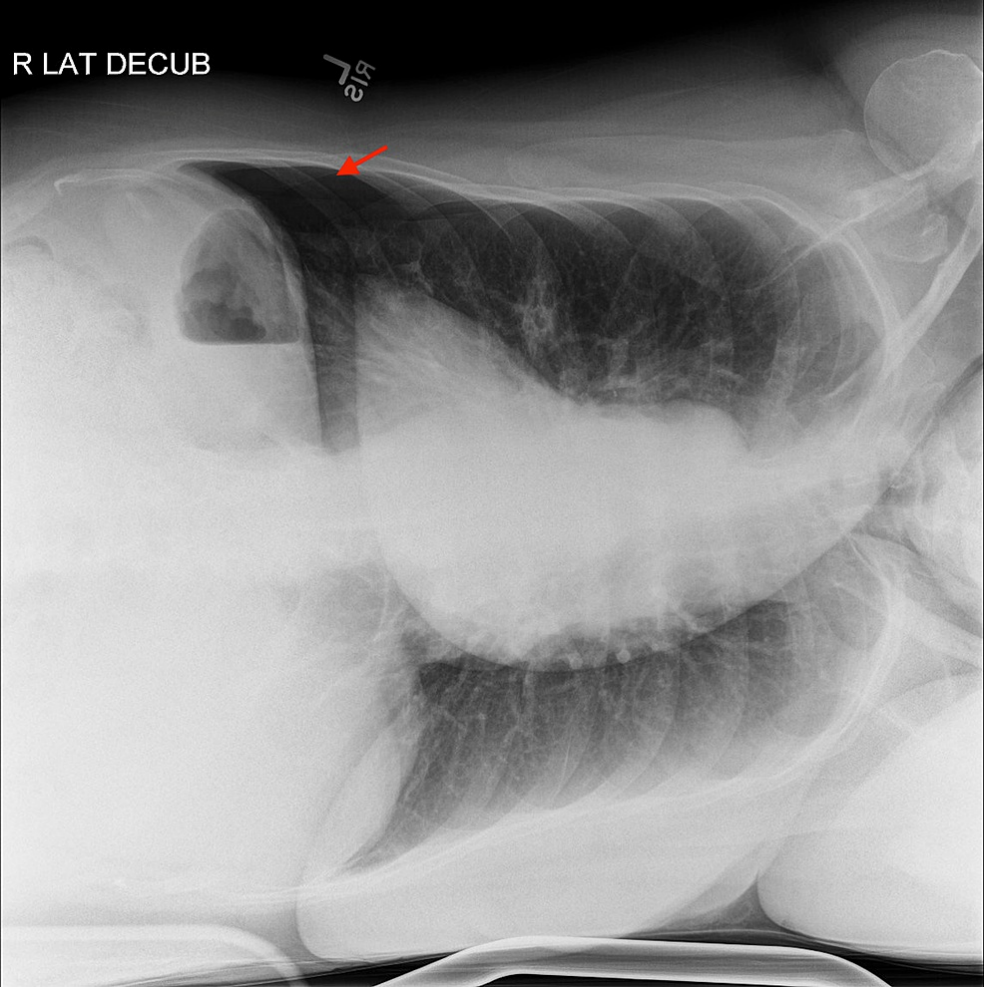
Right lateral decubitus chest X-ray Red arrow - Left pneumothorax
